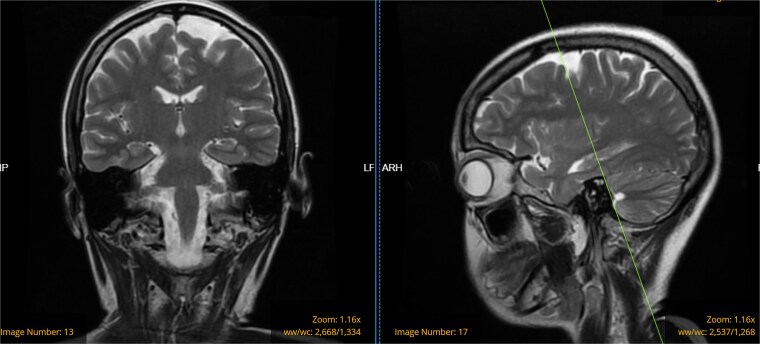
MRI T2 flair (a) axial and (b) coronal showing mild bi-frontal atrophy.
